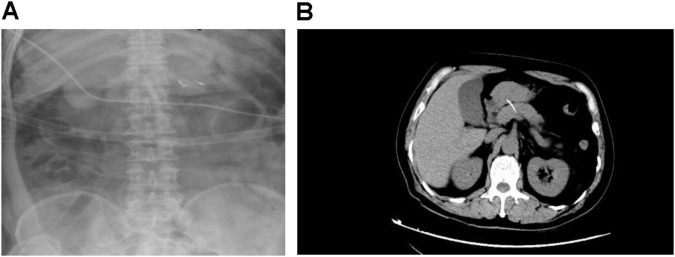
Representative X-ray (A) and computed tomography (CT) (B) images of pancreatic fishbone impaction.
